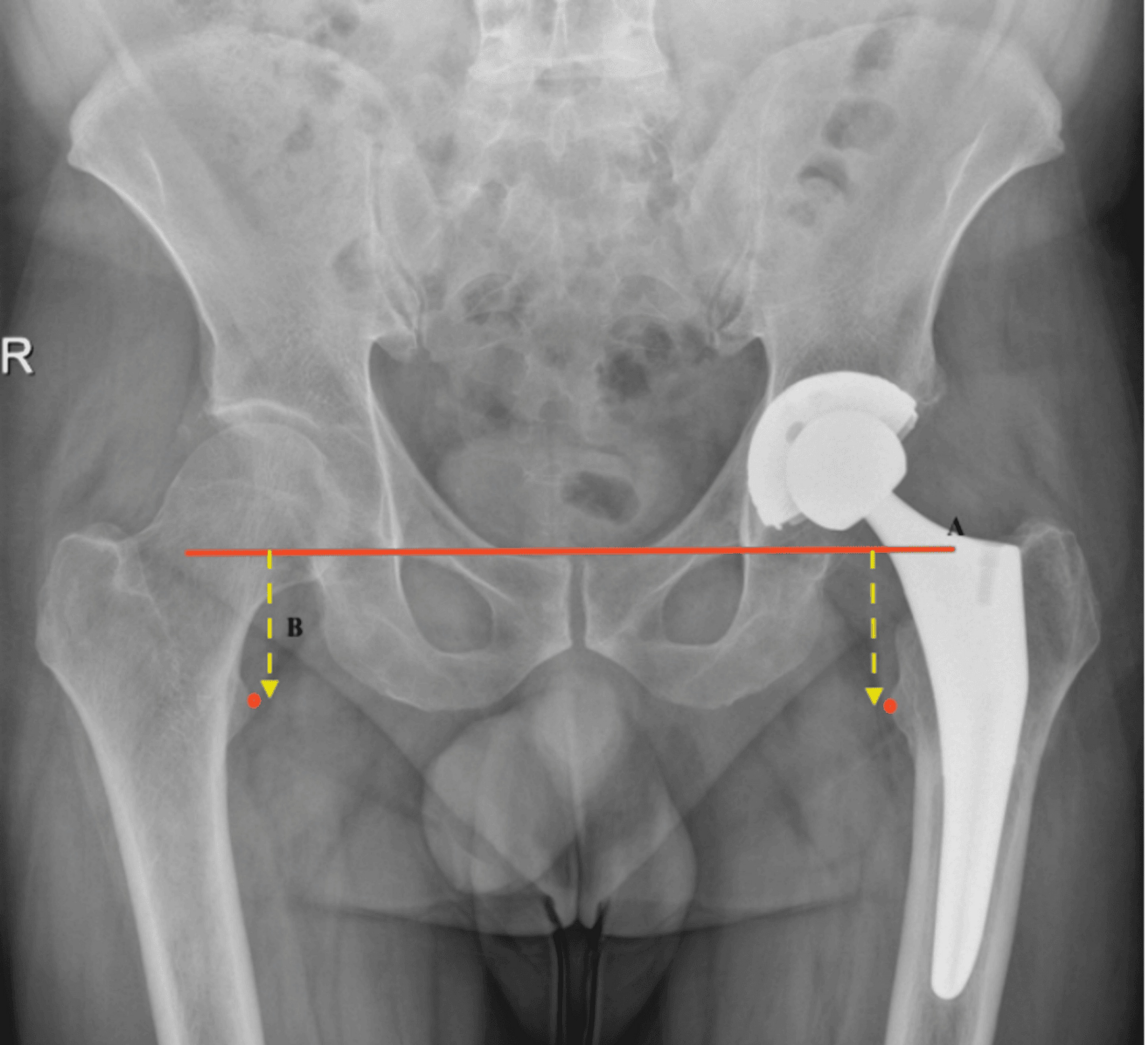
LLD measurement from AP view X-ray of the pelvis. (A) The inter-teardrop line. (B) The distance between the inter-teardrop line and the most prominent portion of the lesser trochanter. LLD = leg length discrepancy; AP = anteroposterior
